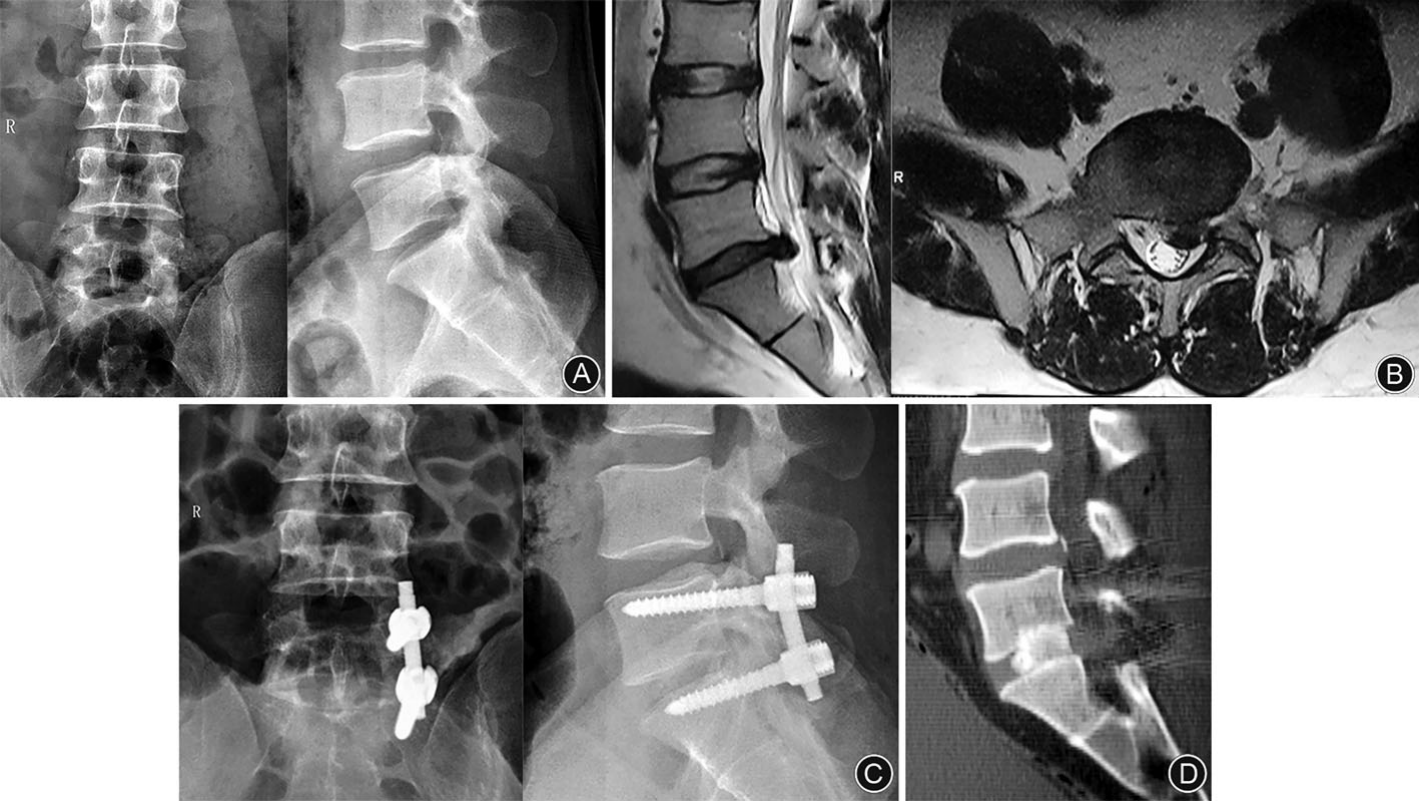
Clinical imaging from one representative patient (male, 56 years old) who complained of lumbago and left lower limb extremity pain for approximately 6 months. (A) X‐ray images of lumbar vertebrae anteroposterior and lateral position plain films showed no vertebral olisthy. (B) T2‐weighted sagittal (left) and axial (right) preoperative magnetic resonance images (MRI) of the lumbar spine showed the L_5_–S_1_ disc herniation. (C) The X‐ray imaging indicated that the location of the internal fixator was excellent. (D) The lumbar vertebrae CT indicates that the location of the grafting bone block with intervertebral space was fine, and the synostosis of intervertebral space was also excellent.
